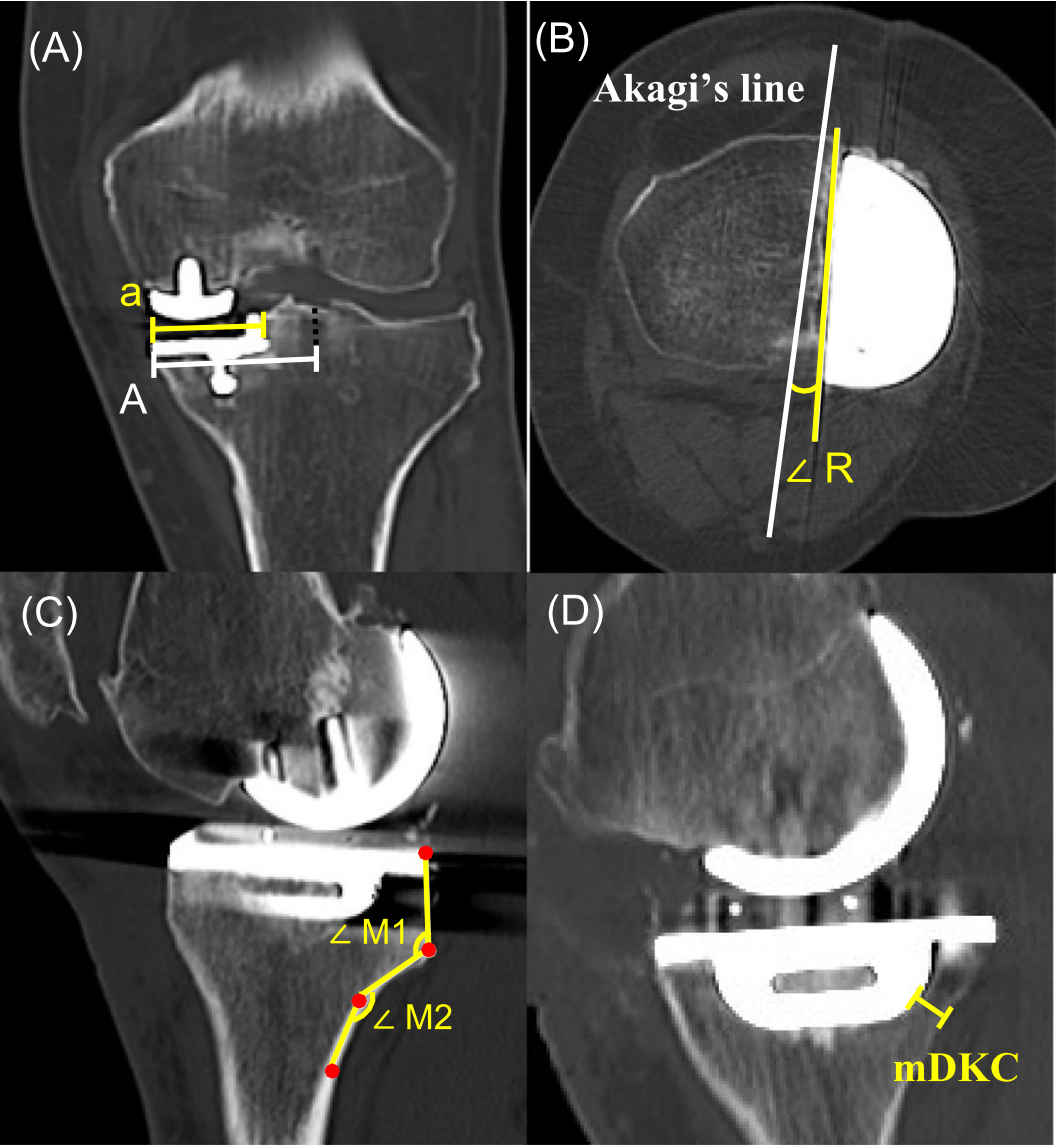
Radiographic measurement of tibial component position. a The mediolateral tibial component position was measured and is represented as ratio a/A, where, “a” is the distance from the medial edge of the proximal tibia to the lateral wall of the tibial component and “A” is the distance from the medial edge of the proximal tibia to the tibial axis. b The axial alignment of the tibial component was assessed in terms of ∠ R; the angle between a line tangential to the lateral wall of the tibial component and Akagi’s line. Values indicative of external rotation of the tibial component relative to Akagi’s line were considered to be positive. Akagi line is referred to a line connecting the middle of the posterior cruciate ligament and the medial border of the patellar tendon attachment. c The posterior cortex of the proximal tibia had two obvious angles with the 90° rotated “Z”-shape. From proximal to distal, the angles were known as the first and second angles, respectively, and were recorded as ∠ M1 and ∠ M2, respectively. All the angles were measured and expressed as acute angles for statistical convenience. d The minimum distance between the tibial component keel and the outer margin of the posterior tibial cortex was recorded as mDKC
